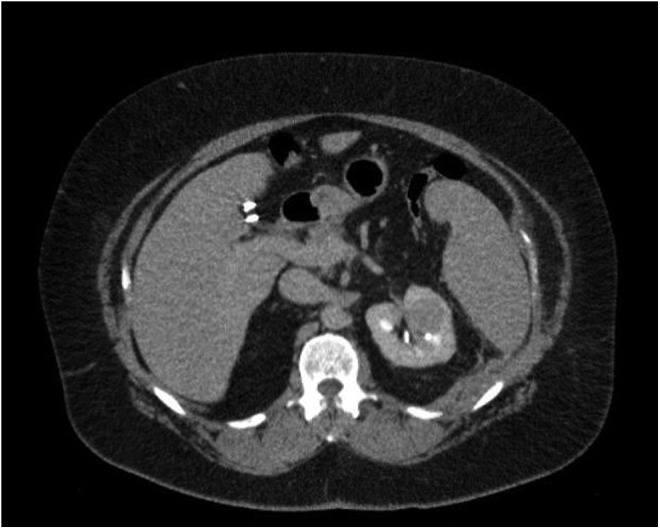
CT scan demonstrating 3 cm enhancing left renal mass.
